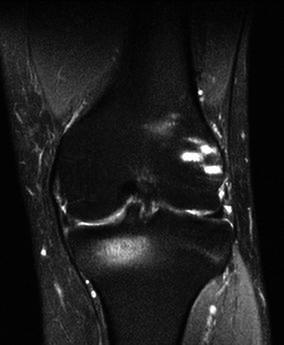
Post operative MRI scan showing intact ACL and healed medial meniscus (coronal view)
